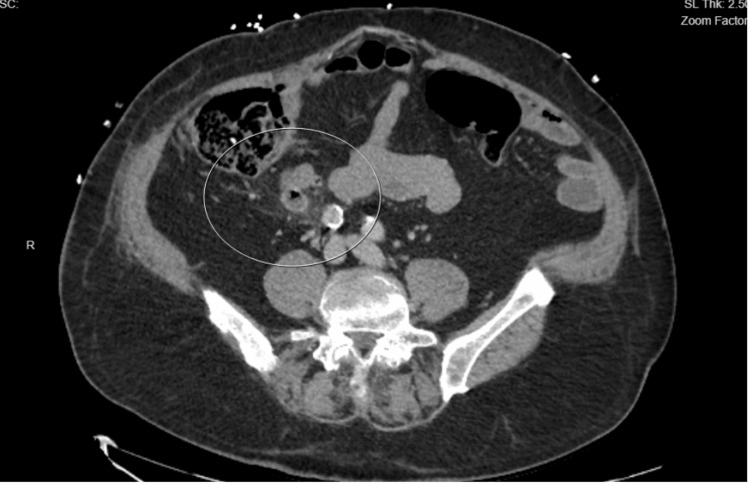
CT scan of diverticulitis of terminal ileum CT, Computed Tomography
